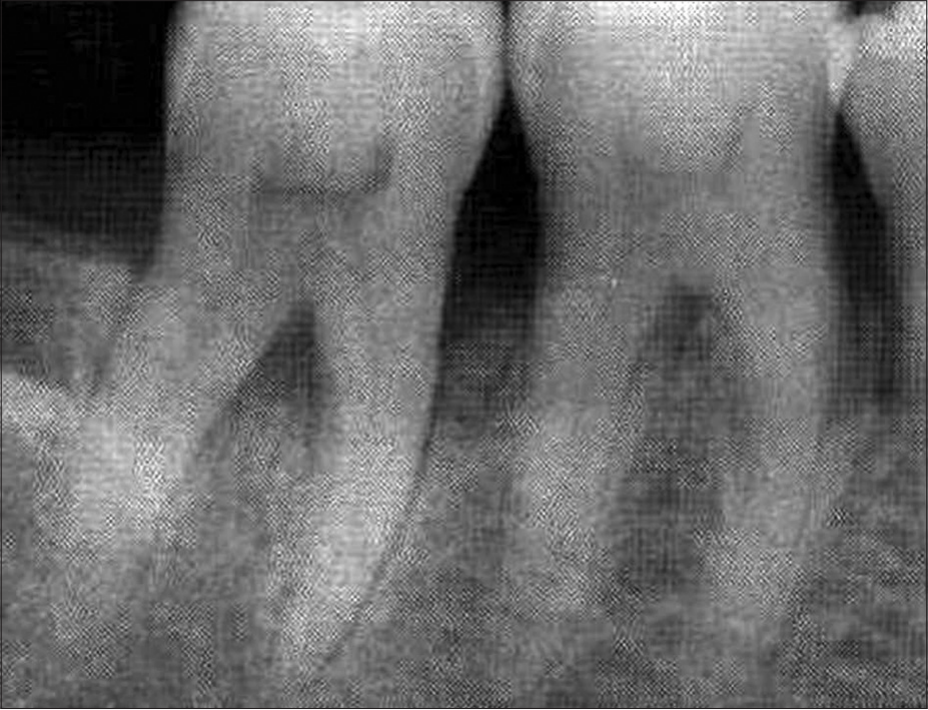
Radiograph of experimental site at baseline
